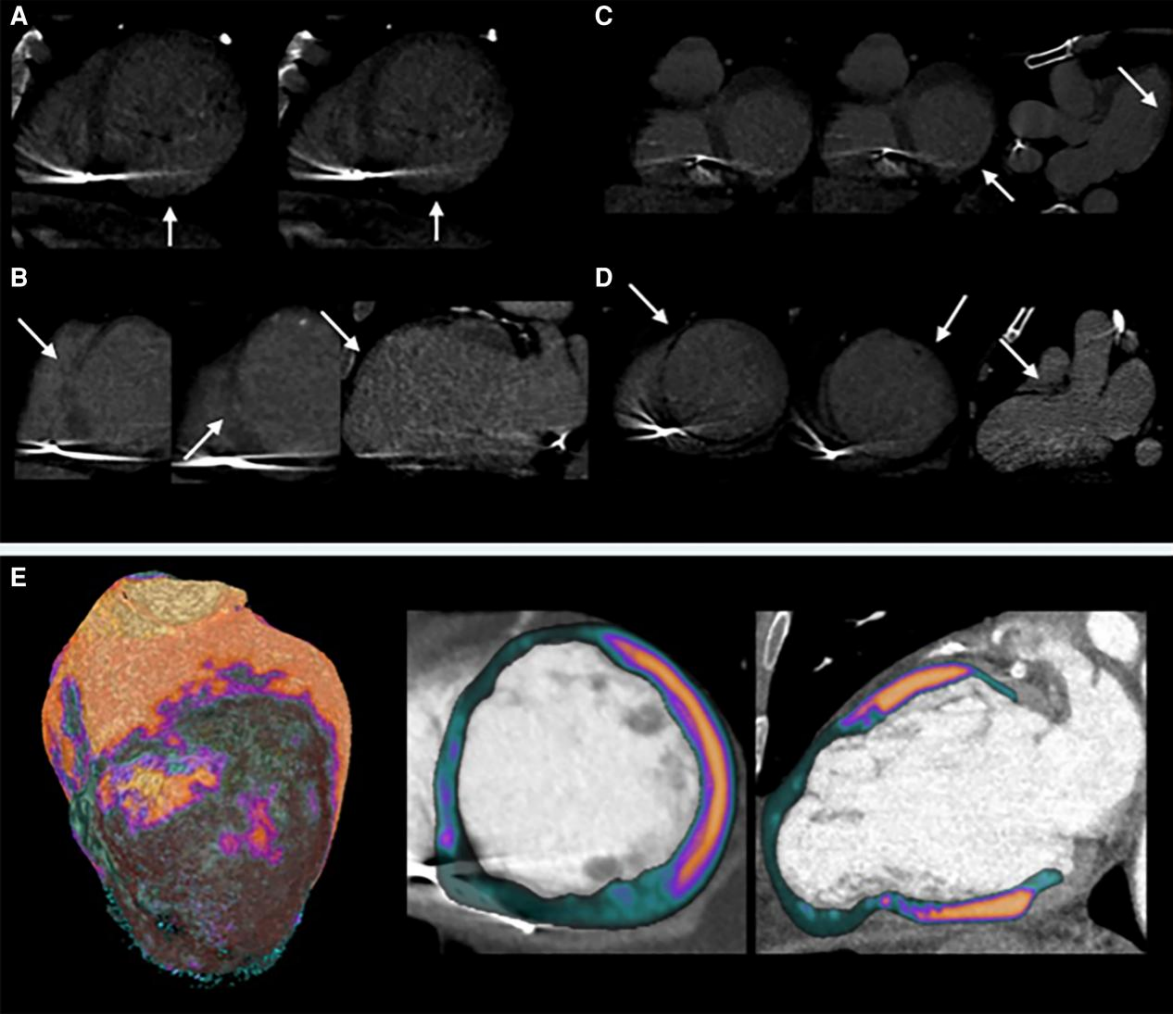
Late-iodine enhancement cardiac computed tomography showing: (*A*) Transmural late enhancement in the inferior wall in #Patient 1. (*B*) Transmural late enhancement in the mid-apical anterior wall and in the septum in #Patient 3. (*C*) Non-ischaemic late enhancement in the infero-lateral wall in #Patient 4. (*D*) Transmural late enhancement in all apical segments and in the mid antero-septal and antero-lateral wall in #Patient 5, Panel *E*. Arterial-phase computed tomography images of #Patient 5, reviewed with a resting myocardial perfusion assessment software, showing a thin-walled apical aneurism and an extensive area of hypoperfusion involving the mid-apical antero-septal and antero-lateral wall, periapical segments, and true apex.
